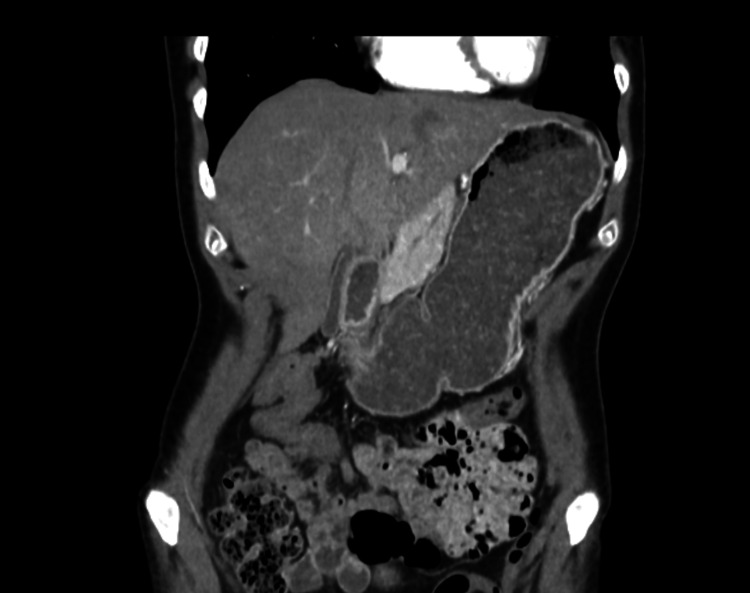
Coronal CT image showing a marked dilated stomach CT: Computed Tomography
